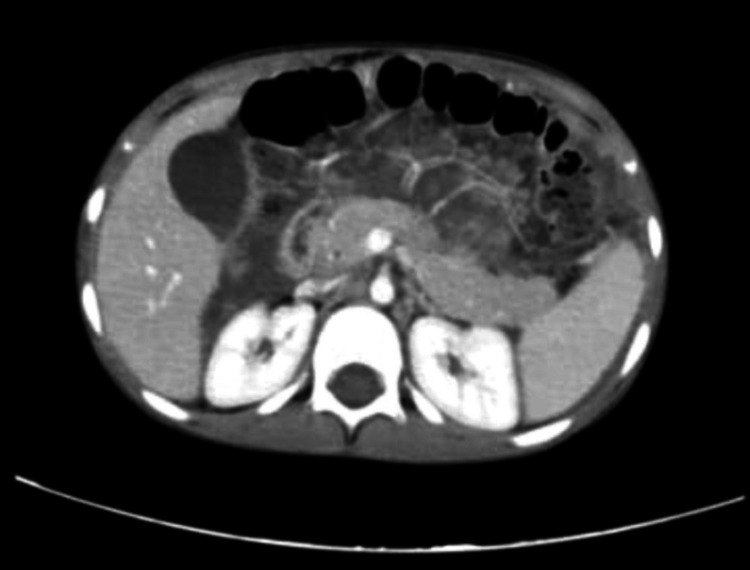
Axial plane of repeated CT scan of second Emergency Department presentation.
